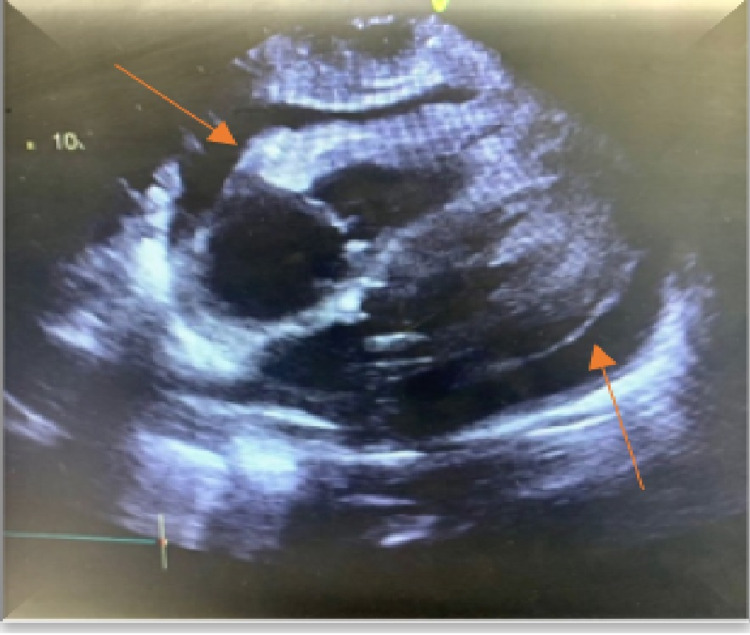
Two-dimensional echocardiography apical four-chamber view showing pericardial effusion.
